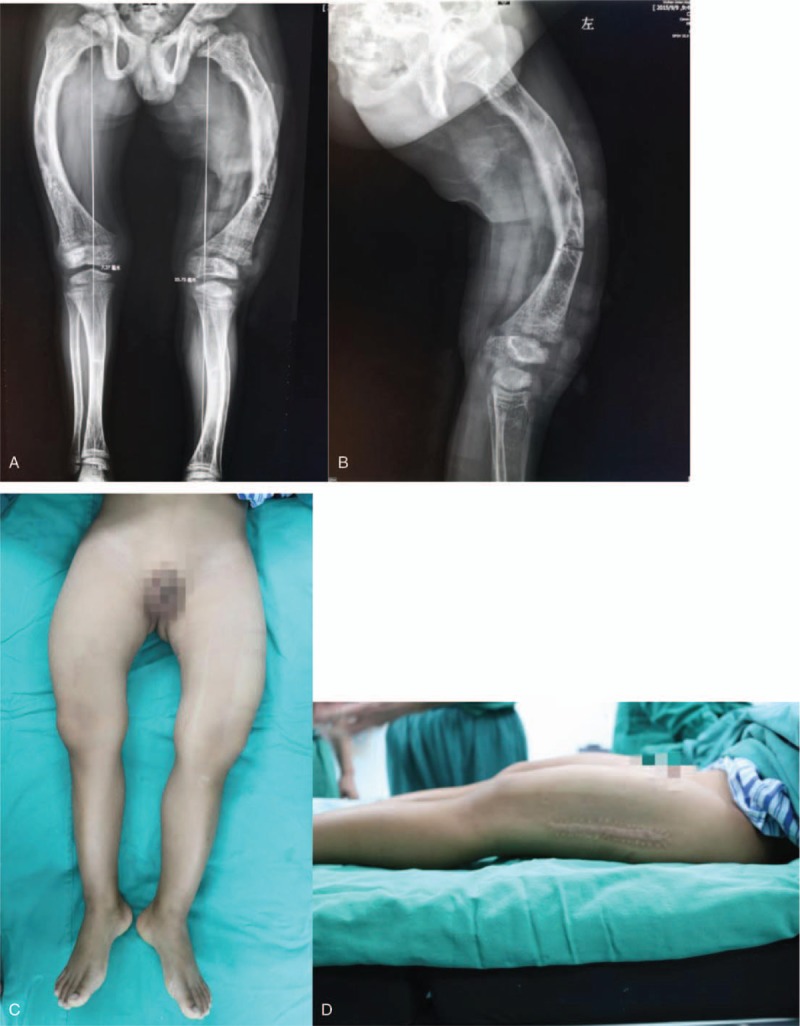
(A) Full-length lower extremity radiograph of 11-year-old boy of left distal femoral fracture; (B) lateral view of femur; (C) appearance of lower extremities on supine position; and (d) lateral view of thigh.
